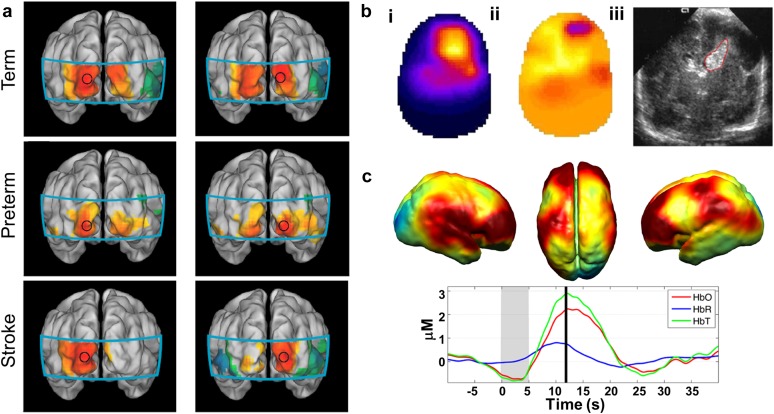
Application of DOT in studies of neonatal brain injury. (a) Maps of resting state functional connectivity using HD-DOT (fcDOT). A seed placed in left or right visual cortex reveals strong temporal correlations throughout contralateral visual cortex in term born neonates. Infants born preterm have weaker bilateral connectivity within the visual cortex. Bilateral visual network connectivity was completely absent in a preterm infant with a unilateral left occipital stroke. [Reproduced with permission from White *et al.*, NeuroImage 59, 2529–2538 (2012). Copyright 2012 Elsevier.] (b) Whole brain reconstruction of time domain optical data acquired from a preterm neonate with left-sided intraventricular hemorrhage (IVH). Regional blood volume (i), regional oxygen saturation (ii), and cranial ultrasound demonstrate disruptions in oxygenation and hemoglobin concentration in the region near the hemorrhagic parenchymal infarct (iii). [Reproduced with permission from Austin *et al.*, NeuroImage 31, 1426–1433 (2006). Copyright 2006 Elsevier.] (c) Whole brain DOT cortical reconstruction corresponding to the peak total hemoglobin concentrations following 4–6 s long EEG burst activity on a background of a burst suppressed EEG in an infant with grade III hypoxic ischemic encephalopathy (HIE). [Adapted with permission from Chalia *et al.*, Neurophotonics 3, 031408 (2016). Copyright 2016 SPIE.]
